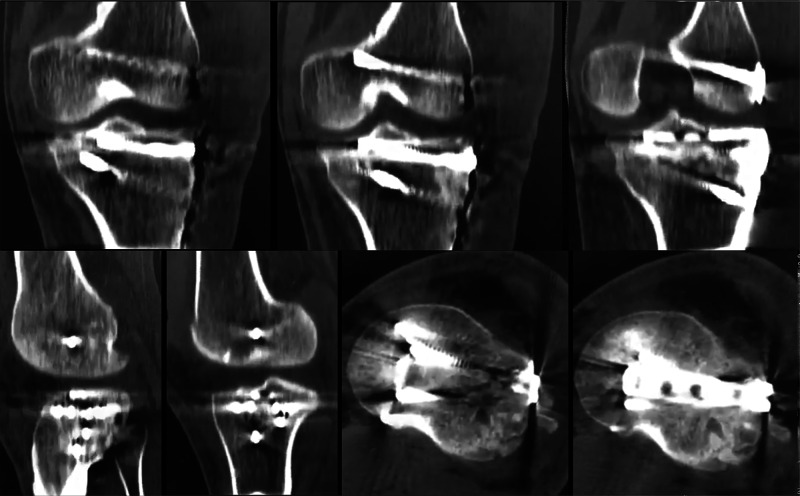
GM, 35-year-old, male patient, fall from a motorcycle Postoperative CT scan of the left knee at 24 months CT: computed tomography
